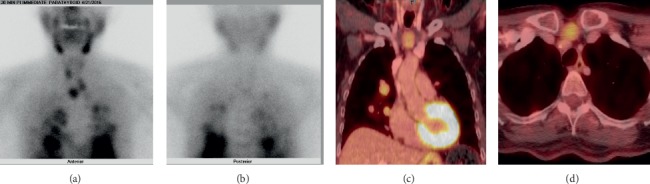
Parathyroid carcinoma. A 68-year-old female presents with recurrent hyperparathyroidism due to recurrent parathyroid carcinoma. Planar anterior (a) and posterior (b) sestamibi scintigraphy at 20 minutes and ^18^F-fluorodeoxyglucose (^18^F-FDG) hybrid positron emission tomography and computed tomography (PET/CT) show multiple marked FDG-avid lesions (c, d) attributable to both local recurrence and multiple local and lung metastases.
